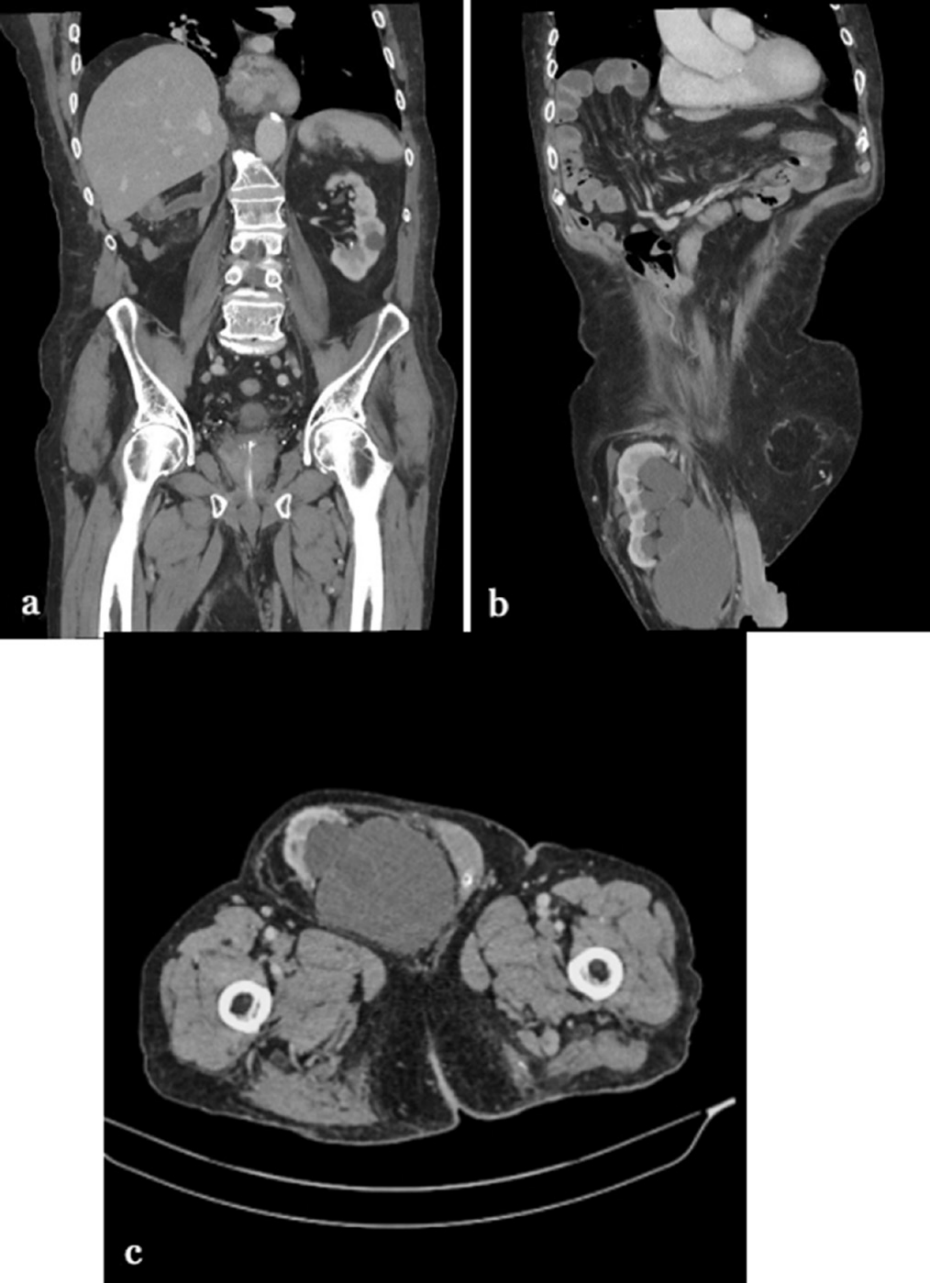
CT scan from 2021 showing (a) empty right renal fossa on a coronal slice (b) sagittal view demonstrating the right kidney in the right hemiscrotum with evidence of hydronephrosis along with moderate fluid in the hernial sac (c)the right kidney at the level of the proximal right femur.
